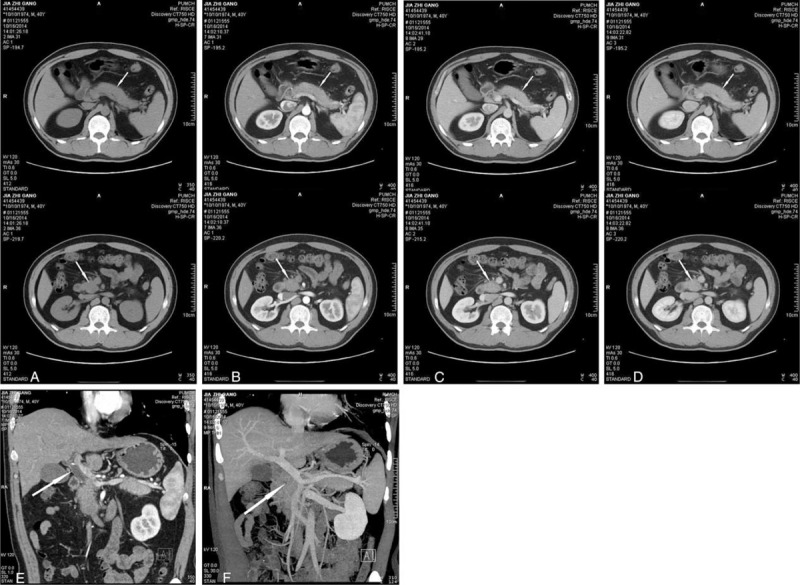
Pancreatic protocol with CT + 3D reconstruction demonstrates an uncinate mass of the pancreas and the swollen in the body and tail of the pancreas. (A) to (D) represent the plain phase, arterial phase, portal phase, and venous phase, respectively. (E) shows the dilated common bile duct, and (F) reveals the anatomical relationship of the uncinate process and the superior mesenteric vein.
